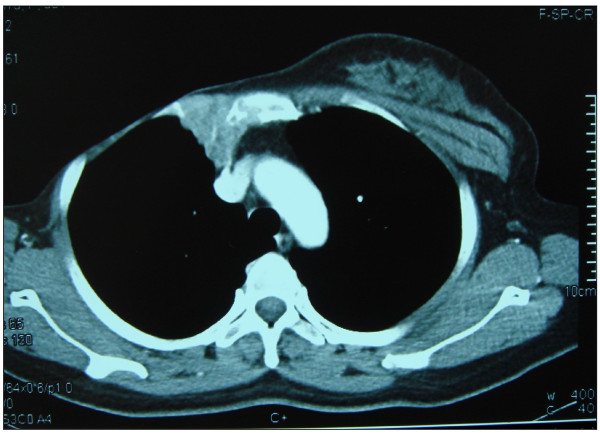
CT image demonstrated internal mammary node forming a mass with a diameter of 3 cm at a level of the second right anterior intercostal space accompany by sternal erosion.
